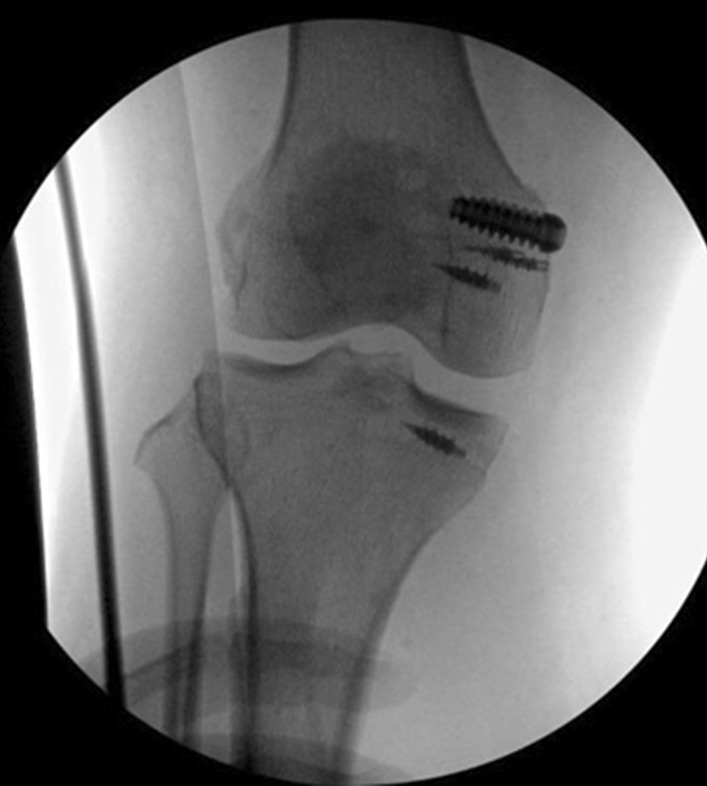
Intraoperative x-rays showing appearance of reconstructed and stable knee following removal of external fixation, anchors *in situ* following medial collateral ligament  repair and medial patellofemoral ligament reconstruction
